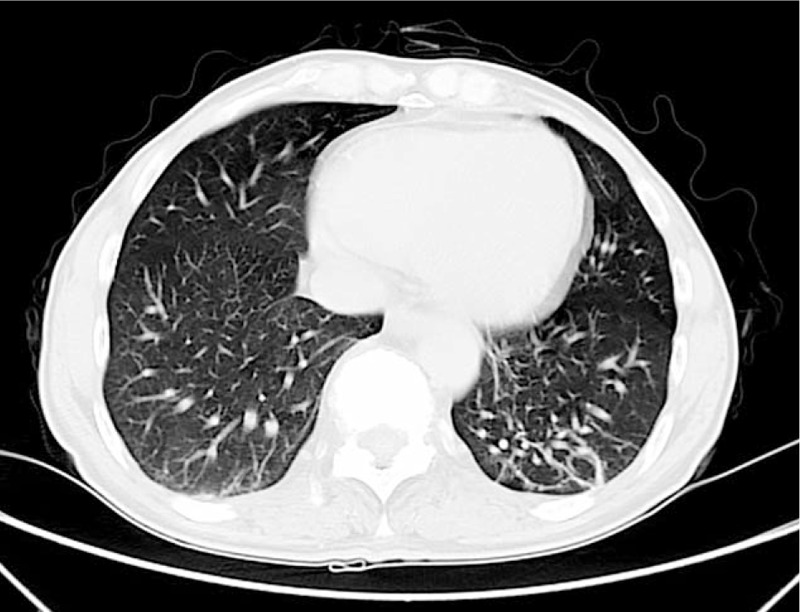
Chest CT scan revealed fibrosis within the irregular strip located in dorsal anasal segment of lower lobes.
